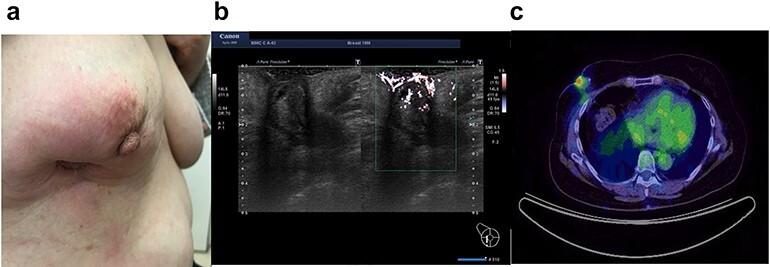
Case 3 presented with erythematous changes in the skin of the right breast (a). Ultrasound evaluation revealed a mixed pattern mass lesion 26.3 × 34.8 × 25.0 mm in the right breast (b), and PET-CT showed a right breast mass with an SUV max of 4.0 (c).
